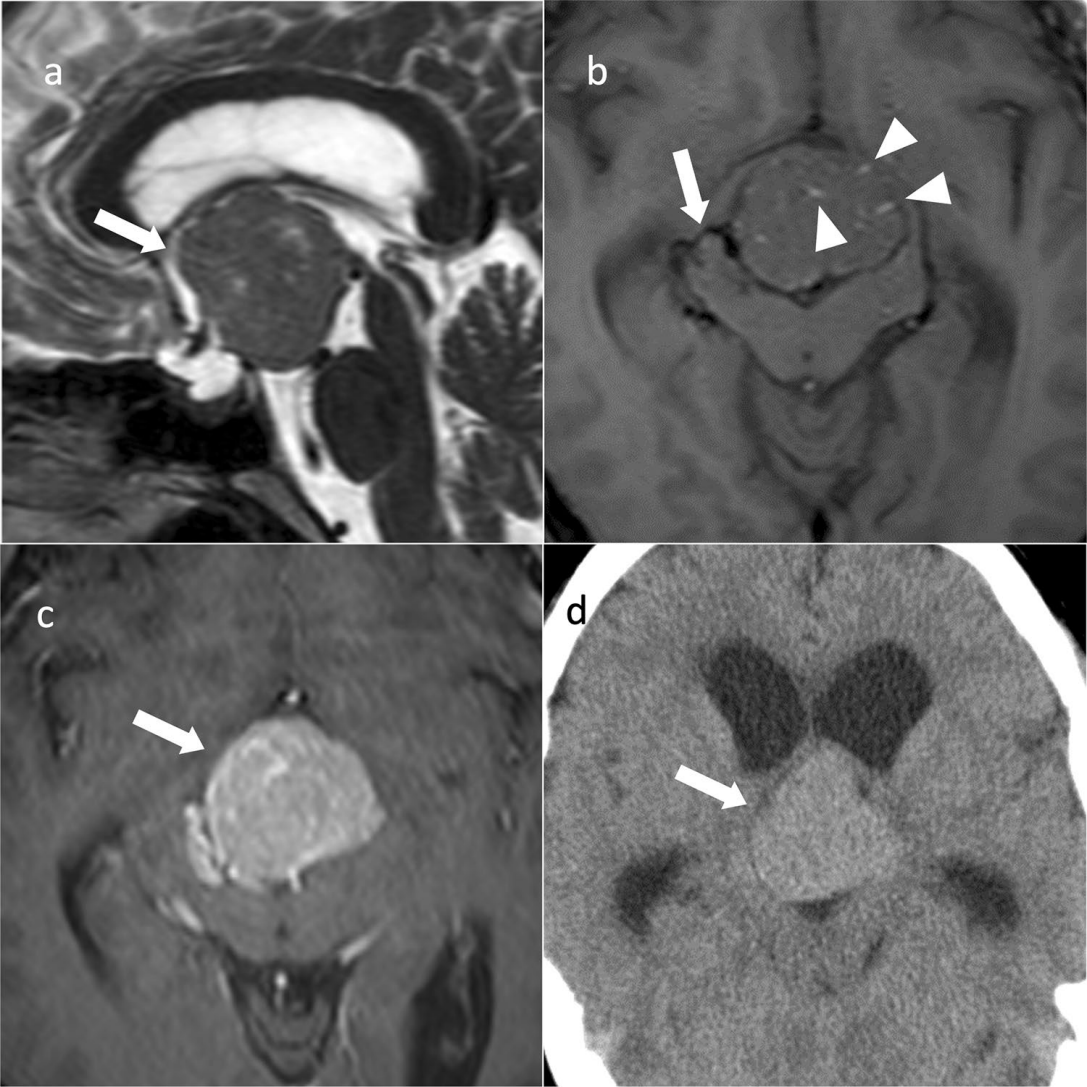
Pituicytoma. An approximately 40-year-old male with mild cognitive decline. a Sagittal T2WI shows a lobulated mass with isointensity compared to gray matter in the suprasellar region (arrow). b Axial T1WI shows a flow void around the mass (arrow). Small hyperintense areas within the mass show slow-flowing vessels (arrowheads). c Contrast-enhanced T1WI shows strong and uniform contrast enhancement (arrow). d Non-contrast CT shows mild high density (arrow). Ventricular enlargement is observed
